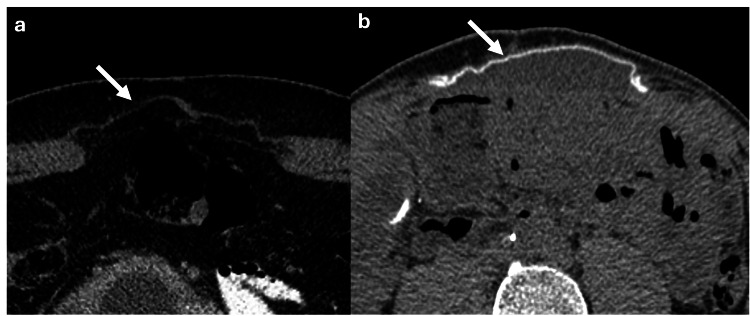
Axial abdominal CT scan without intravenous contrast. (a) Axial CT shows a polypropylene mesh in the anterior abdominal wall that is isodense to the surrounding muscle (white arrow). (b) Axial non contrast CT scan from a different patient with a PTFE mesh in the anterior abdominal wall that is hyperdense to the muscle, allowing better visualization on CT scans (white arrow). The figure was created by the authors.
